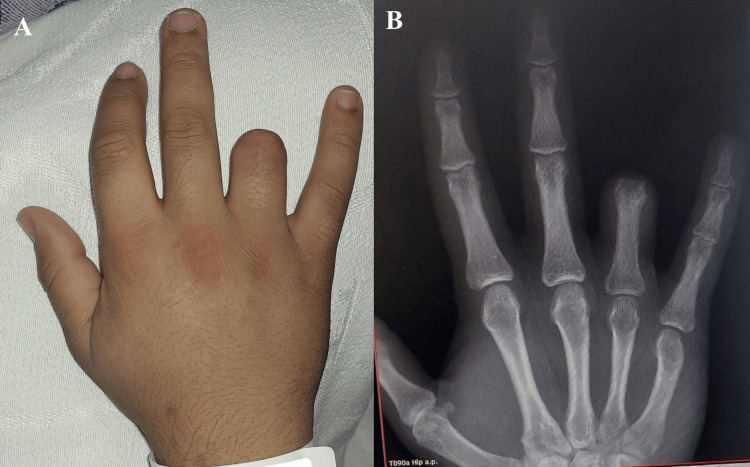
Clinical (A) and radiographic (B) images demonstrating disarticulation at the level of the PIPJ. PIPJ: Proximal interphalangeal joint.
